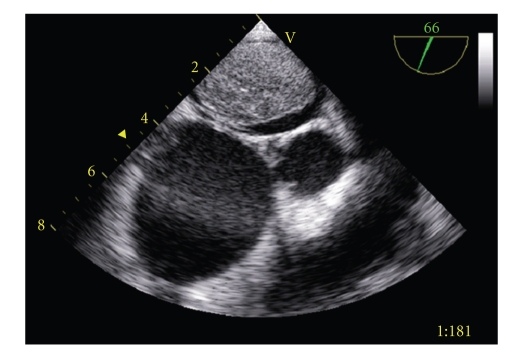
Ultrasound of the heart showing a round left intra-atrial tumour mass, compromising almost the whole left atrium.
